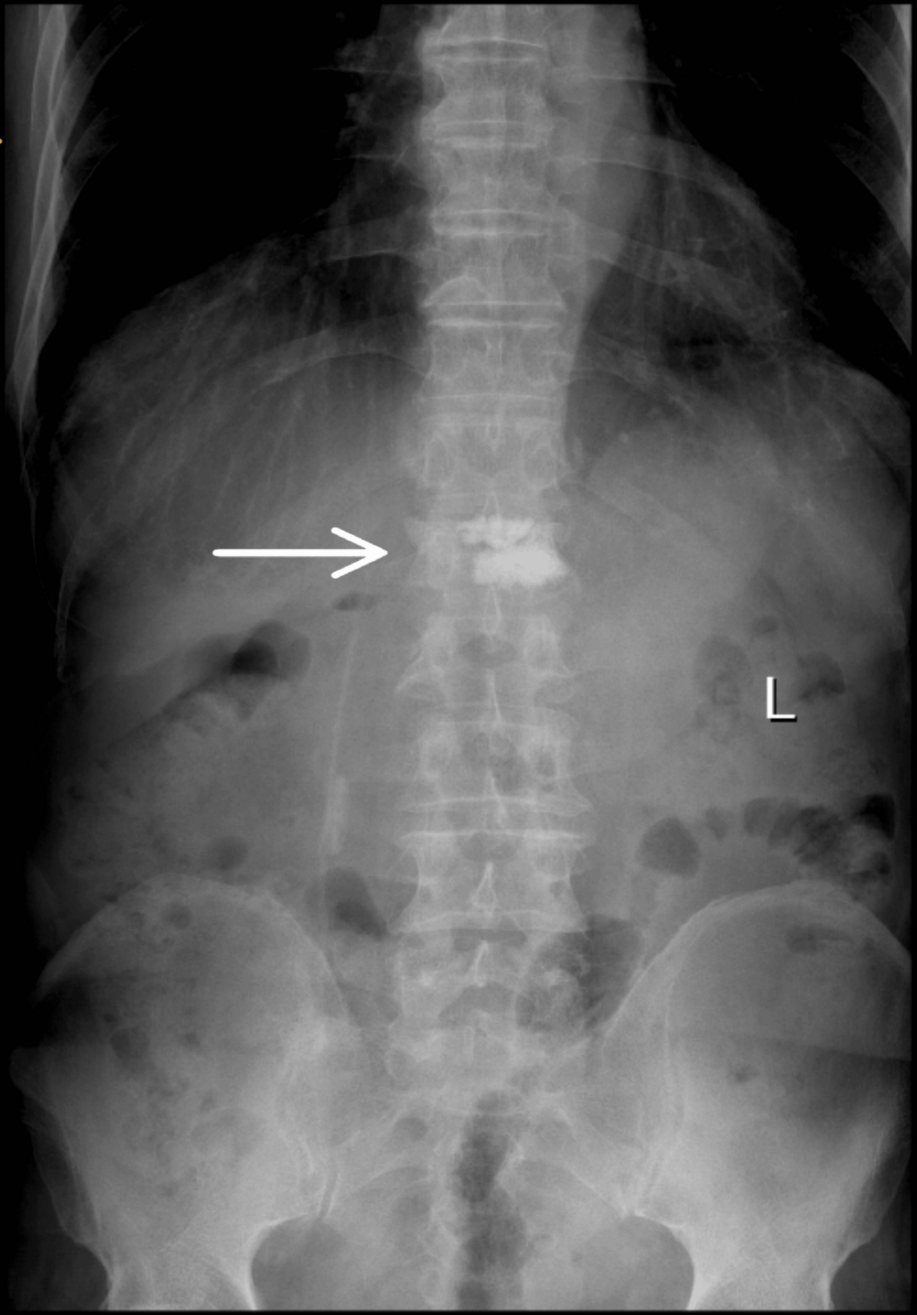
Anteroposterior X-ray view of the L1 vertebra after the surgical procedure.
